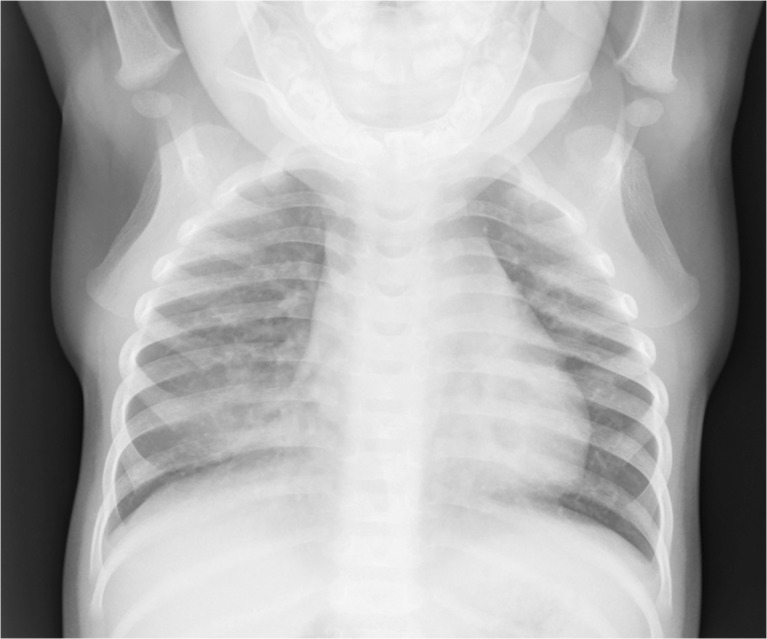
Anteroposterior chest radiograph of an 11 month old male hospitalized with WHO-defined very severe clinical pneumonia demonstrates the silhouette sign with partial loss of the right heart border in the presence of an adjacent opacity, demonstrating endpoint consolidation
